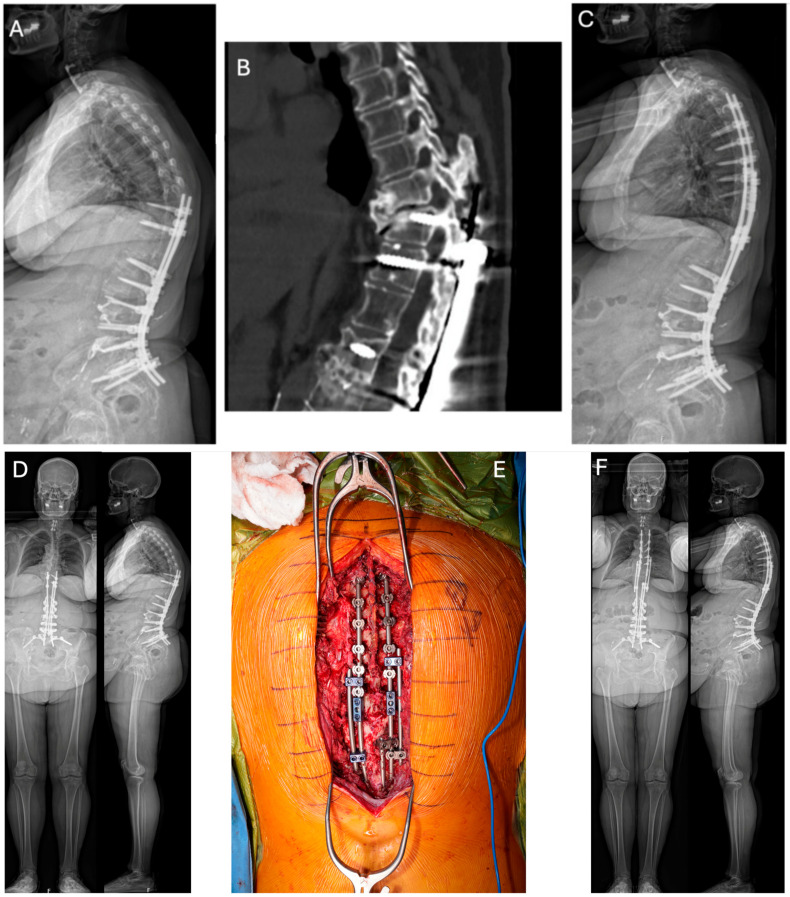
Lateral 36″ radiographs (A,C) and sagittal CT reconstruction (B) of a 58 year-old female with history of combined L1–L5 anterior lumbar interbody fusion and T10-Pelvis posterior spinal fusion, complicated by infection requiring irrigation and drainage, who presented 1 year post-operatively with proximal junctional kyphosis (A,B). She underwent removal of instrumentation, revision posterior spinal fusion from T4 to T11 with Smith-Peterson osteotomy at T9-T10 (C). (D) Pre-operative EOS PA and lateral radiographs demonstrating proximal junctional kyphosis with global sagittal imbalance in the same patient shown in (A,B). (E) (top = cranial; bottom = caudal): Intra-operative clinical photo demonstrating the final construct used to address the proximal junctional failure and restore the sagittal plane following Smith-Peterson osteotomy at T9–T10. End-to-end connectors were utilized to connect the rods placed in the new instrumentation from T4 to T9 with the previously placed instrumentation. Side-to-side connectors were also placed at T8–9 on the left and T7–8 on the right and connected at T12 on both sides. (F) Post-operative EOS PA and lateral radiographs highlighting improved global alignment following removal of instrumentation, revision posterior spinal fusion from T4 to T11 with Smith-Peterson osteotomy at T9–T10.
